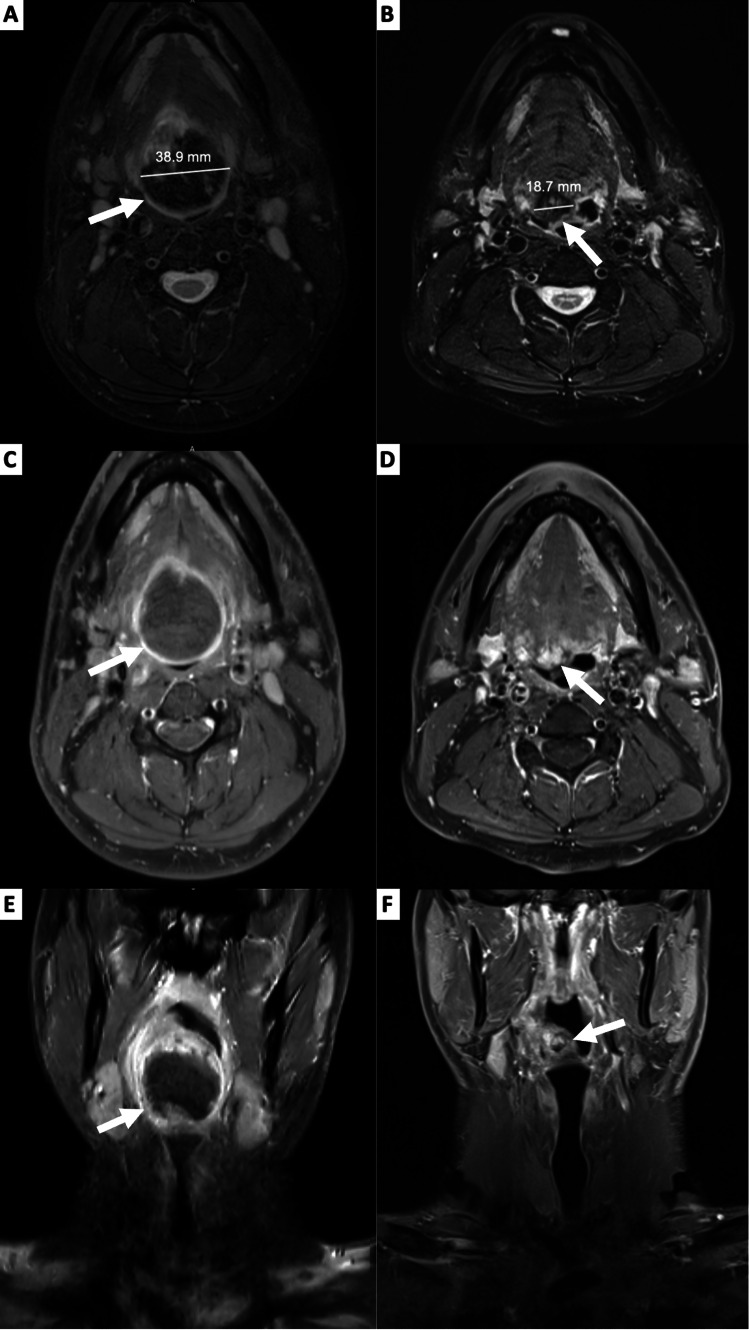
MRI images before and after chemotherapy Panels A, C, and E: The left column shows MRI images before chemotherapy. Panels B, D, and F: The right column shows MRI images after chemotherapy. (A and B) Axial T2 fat saturation images, (C and D) axial T1 fat saturation post-gadolinium contrast images, and (E and F) coronal T1 fat saturation post-gadolinium contrast images. The pretreatment images show a heterogenous mass located in the right paramedian base of the tongue with thickened peripheral enhancement (~3.9 cm x 4.4 cm). The postchemotherapy images show a smaller mass with heterogenous internal enhancement (~1.8 cm x 1.8 cm).
